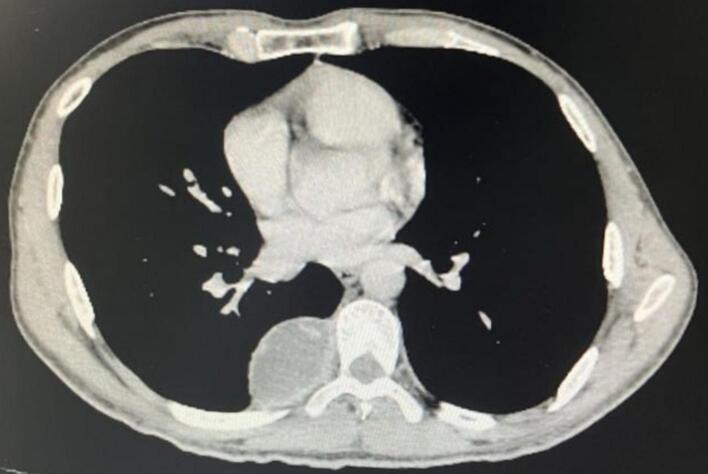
CT scan, mediastinal window showing the posterior mediastinal mass.
